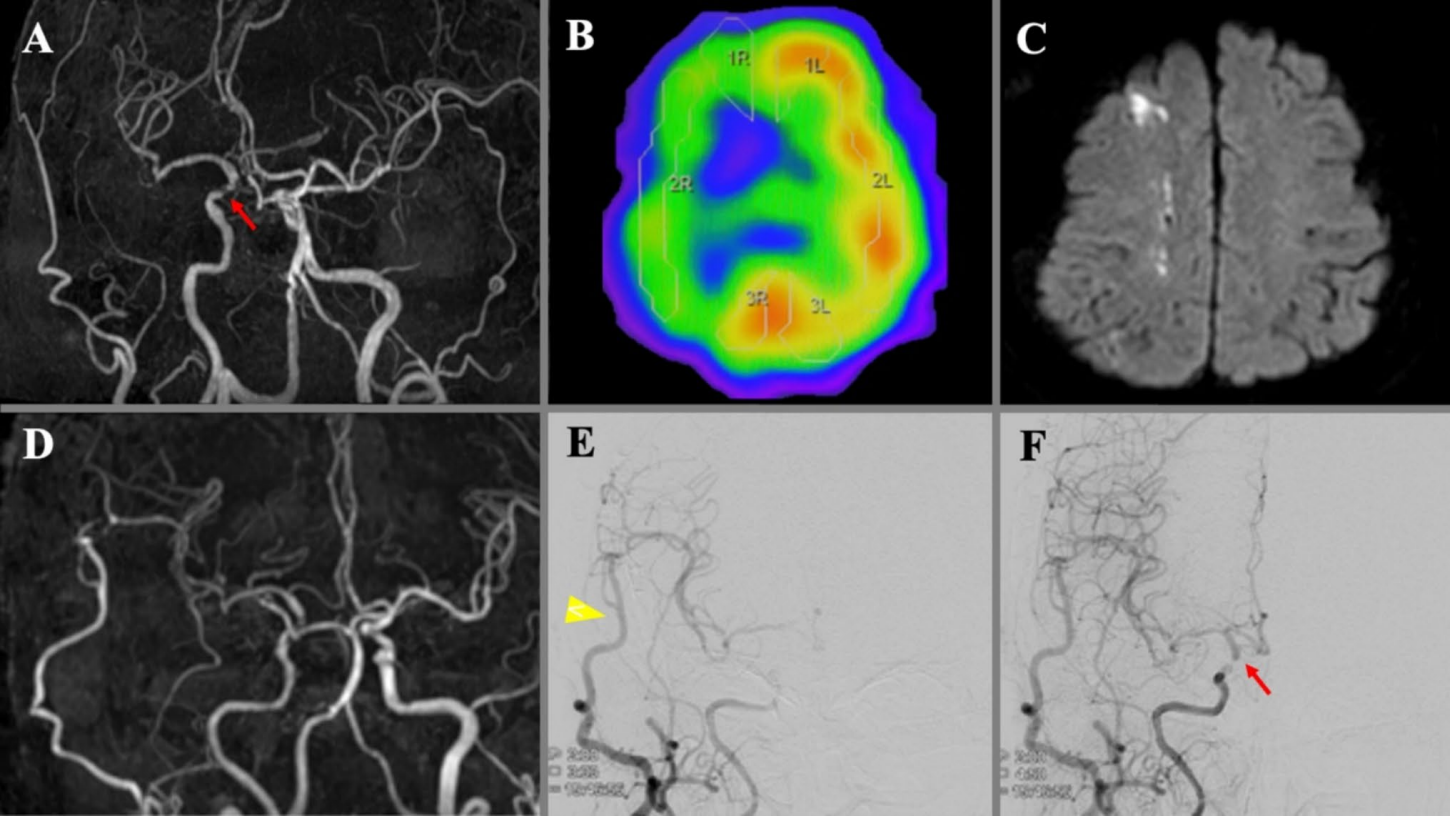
A Preoperative MRA showing severe stenosis of the paraophthalmic segment of the right ICA (red arrow). B Preoperative SPECT demonstrating markedly reduced cerebral blood flow and cerebrovascular reactivity in the affected MCA territory. C, D Diffusion-weighted MRI (C) on postoperative day 3 revealing a new infarction in the right watershed region, despite good bypass patency on MRA (D). E, F Postoperative digital subtraction angiography in the early (E) and late (F) arterial phases showing that the bypass artery (arrowhead) supplied the entire MCA territory. Anterograde flow from the right ICA terminated at the site of severe stenosis (red arrow), and perfusion of the anterior cerebral artery territory was delayed
